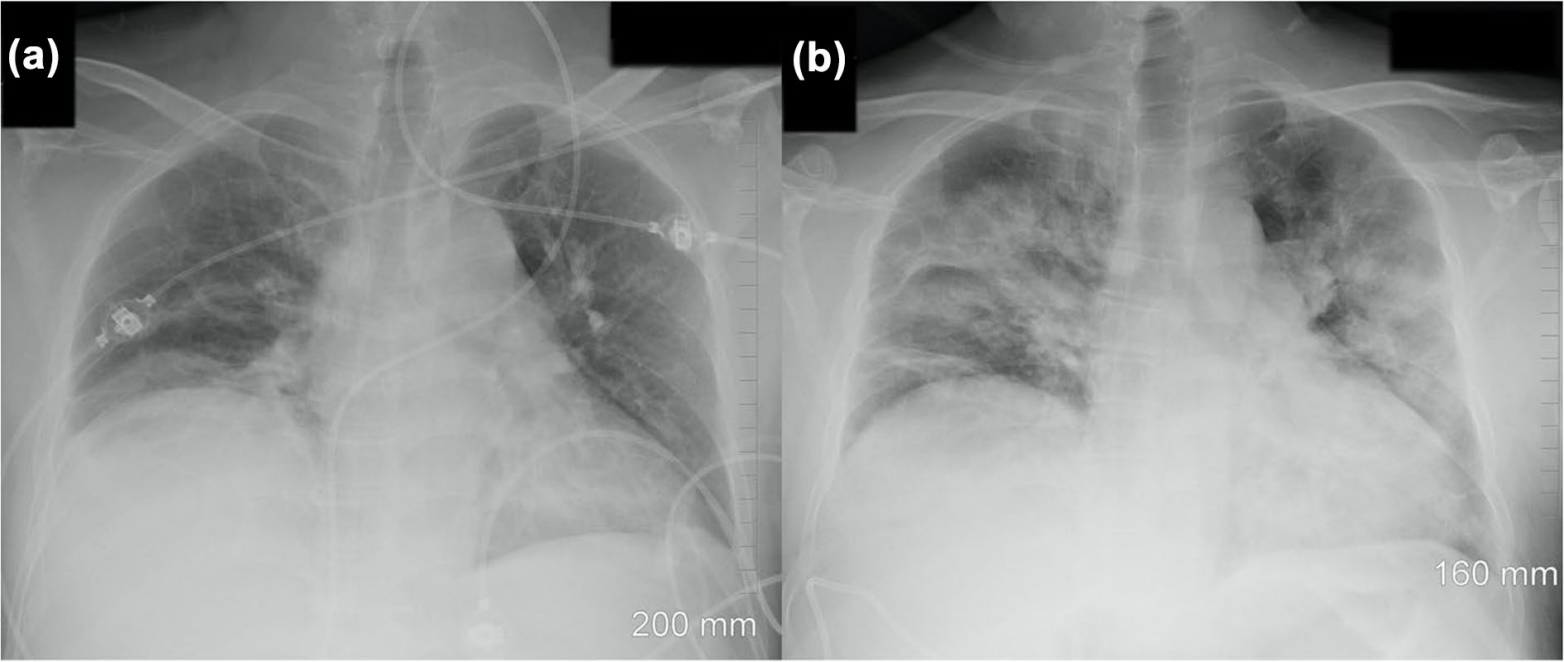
Chest radiographs demonstrate rapidly increasing alveolar lung infiltrates bilaterally. On December 2nd, the chest X-ray a shows mild basal lung infiltrates that progress significantly on December 4th b to extensive bilateral consolidations
